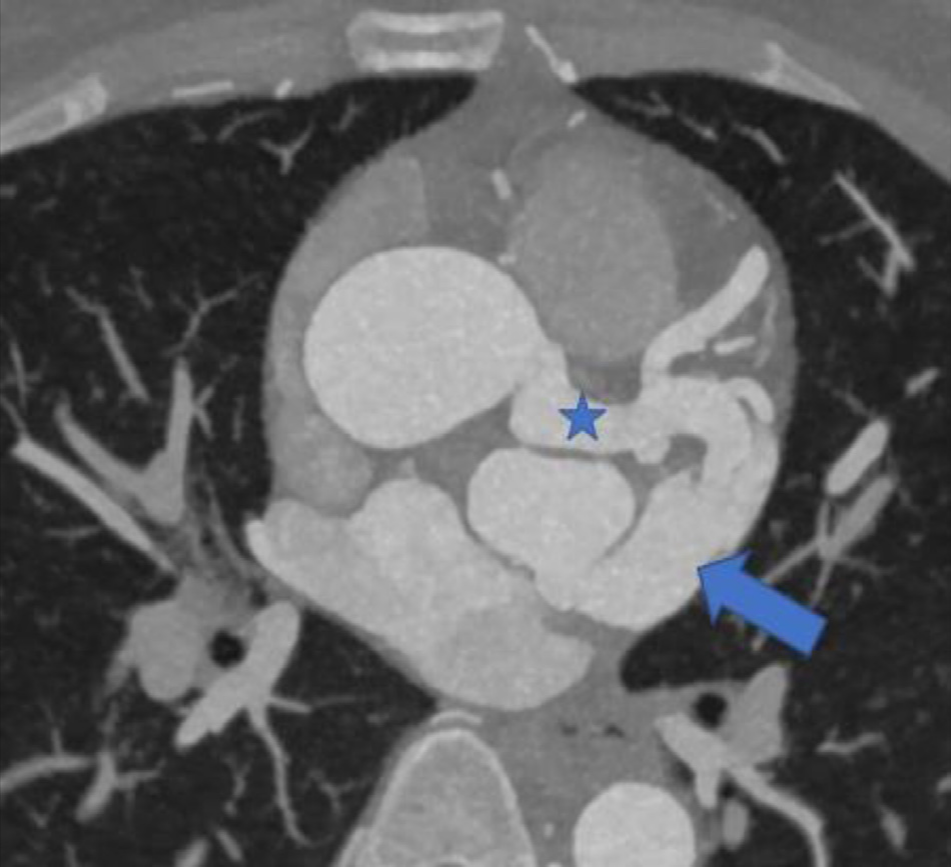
Axial contrast-enhanced CTA maximum intensity projection image: the proximal portion of the LCX CAF (blue arrow) is demonstrated connecting to the dilated left main coronary artery (blue star).
